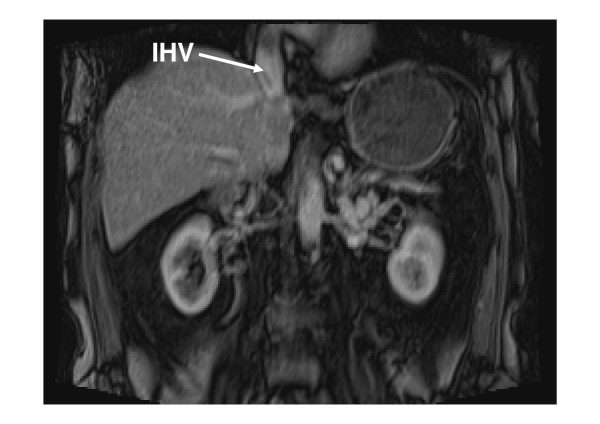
Coronal MRI showing the intrahepatic veins (IHV) empty directly into the right cardiac atrium.
